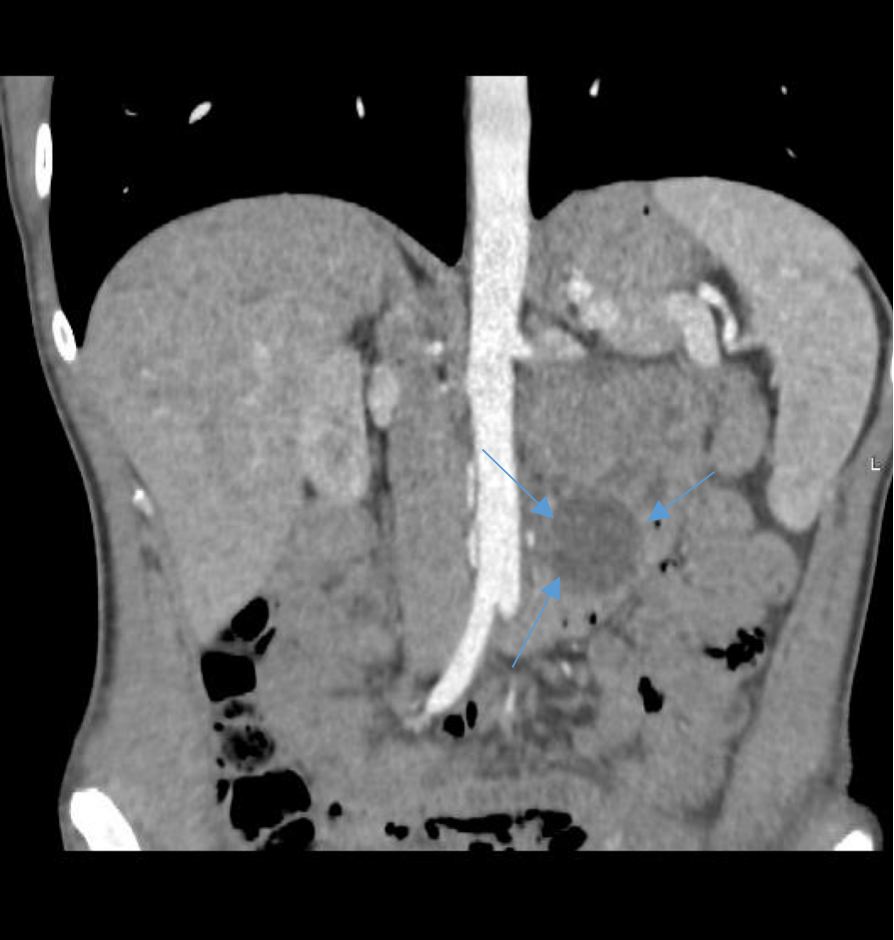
Computed tomography scan of the abdomen. Coronal view – 4.7 × 6.2 × 6.5 cm non-homogenic tumour with a cystic component contacting with an inferior mesenteric vein (blue arrows)
